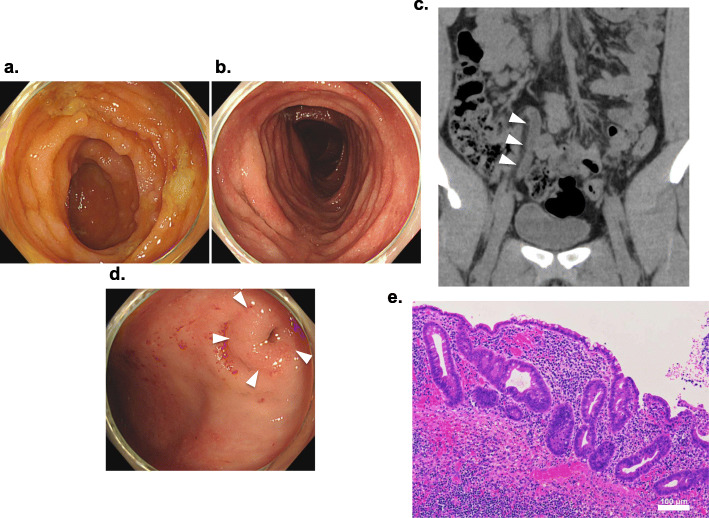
a, b Colonoscopic findings: continuous ulcerative lesions and erythema were observed. c CT fingings: CT revealed a swollen appendix on every symptomatic course (arrow heads). d Colonoscopic findings: The appendiceal orifice was almost normal (arrow heads). e Pathological examination: the pathological finding revealed inflammatory cells infiltration and crypt distortion in the resected appendix
